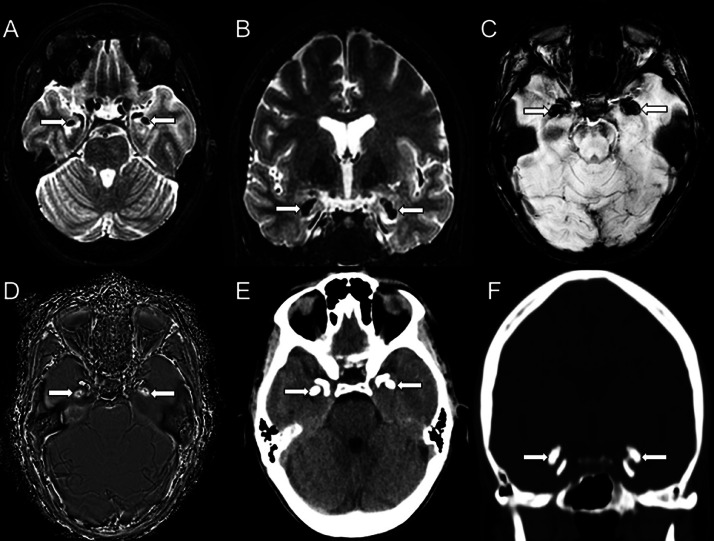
Axial (A), coronal (B) T2-weighted, and SWI (C) images reveal signal loss (arrows) consistent with calcifications on filtered-phase images (D) in the amygdalae and the head of the hippocampi in a 37-year-old female patient with lipoid proteinosis. CT axial (E) and coronal (F) images confirm the presence of calcifications (arrows).
